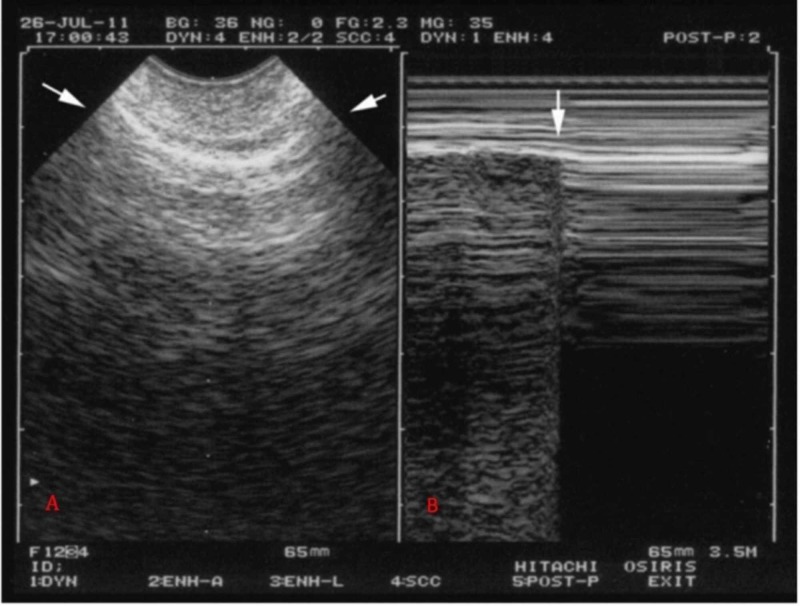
Ultrasound of the third intercostal space along the anterior axillary line The lung point (ultrasound of the 3rd intercostal space along the anterior axillary line). A) Expiration. Absence of lung sliding, plus A-lines; B) M-mode shows the sudden (arrow) inspiratory appearance of the lung point. Permission to use this figure obtained by Lichtenstein et al. [7]
